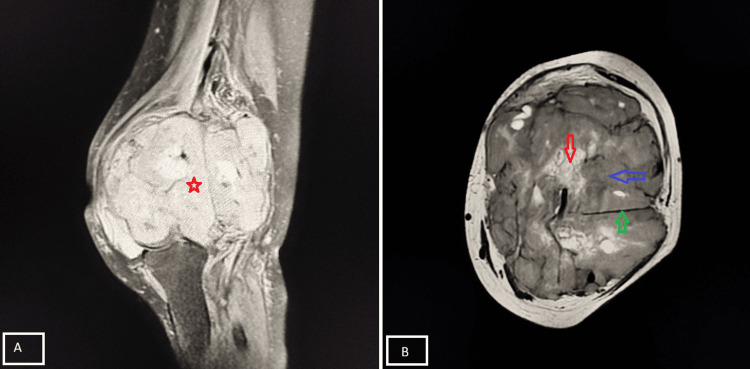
Synovial sarcoma demonstrating the “triple sign” Sagittal PD-FS MRI (A) demonstrates the presence of a heterogeneous multilobulated soft tissue mass (red star). In axial T2-weighted MRI (B), there is the presence of high (red arrow), intermediate (blue arrow), and low (green arrow) signal intensities, representing fluid/necrosis, solid tumor tissue, and fibrous septa, respectively, producing the characteristic “triple sign” of synovial sarcoma. PD-FS, proton density fat-suppressed
